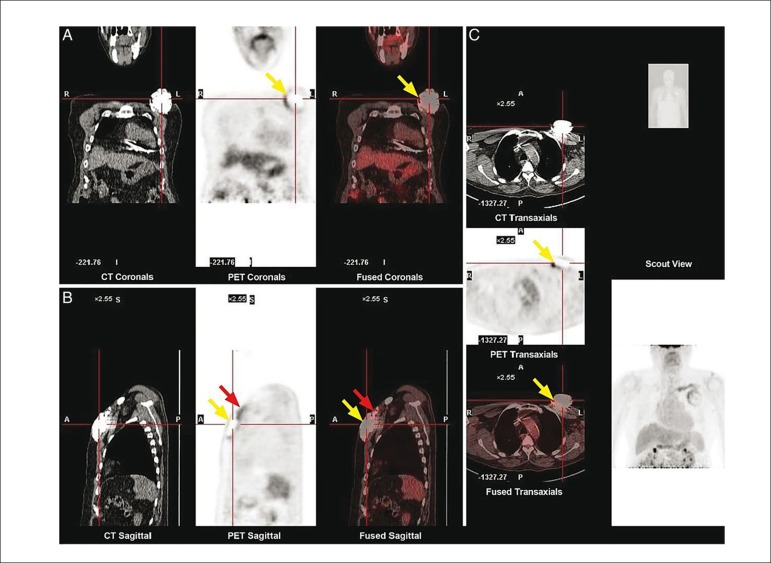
PET-CT in suspected device pocket infection. Example of a positive
